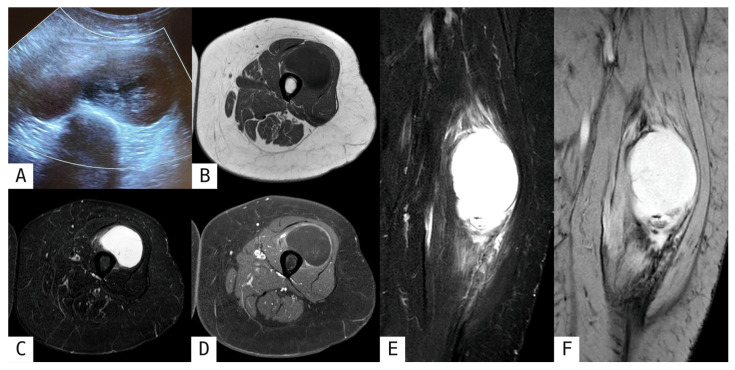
Myxofibrosarcoma of the left thigh. A 67-year-old woman presented with a painless enlarging soft tissue mass in the left thigh one month after a day hike. US transverse scan (A) showed a subfascial heterogeneously hypoechoic mass with smooth margins, with no vascular signals on Power-Doppler. MRI axial T1-weighted (B), STIR (C), T1- weighted FS post-gadolinium (D), coronal STIR (E), and mFFE (F) images showed an oval-shaped lesion in the vastus intermedius with high T2 signal content (C,E,F) and no “blooming” artifact in the gradient-echo sequences (F). The lesion had fusiform margins, was surrounded by muscle oedema and compressed the vastus intermedius and rectus femoris muscles. Resection was performed with tumor-free margins. Pathology from an excisional biopsy demonstrated myxofibrosarcoma.
